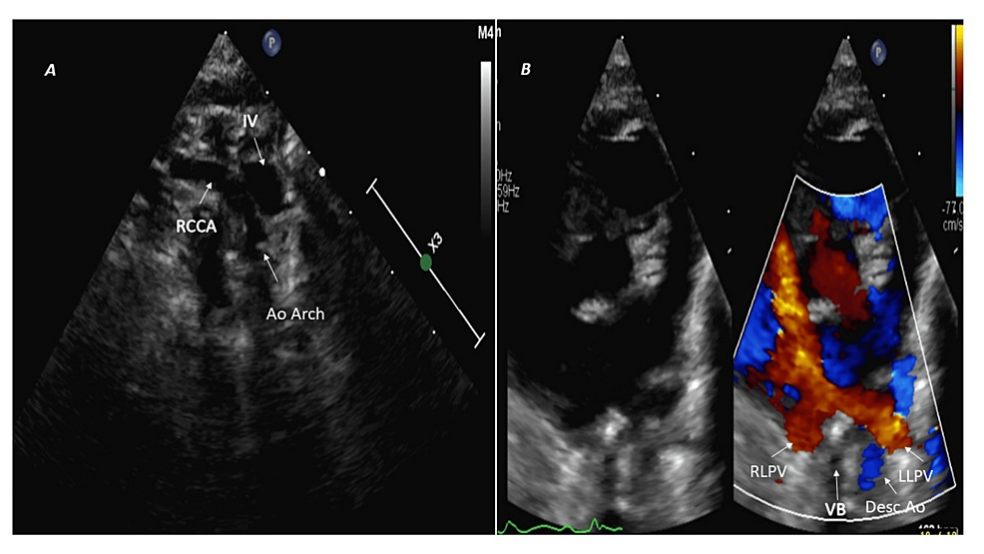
Left aortic arch with aberrant right subclavian artery (A) Suprasternal short-axis view shows the first branch (RCCA) courses toward the right but does not bifurcate. (B) High parasternal short-axis color comparing image: left-sided descending aorta (Desc Ao) located posterior to left lower pulmonary vein (LLPV) on the left side of the vertebral body (VB). Ao Arch: aortic arch; IV: innominate vein; RCCA: right common carotid artery; RLPV: right lower pulmonary vein.
